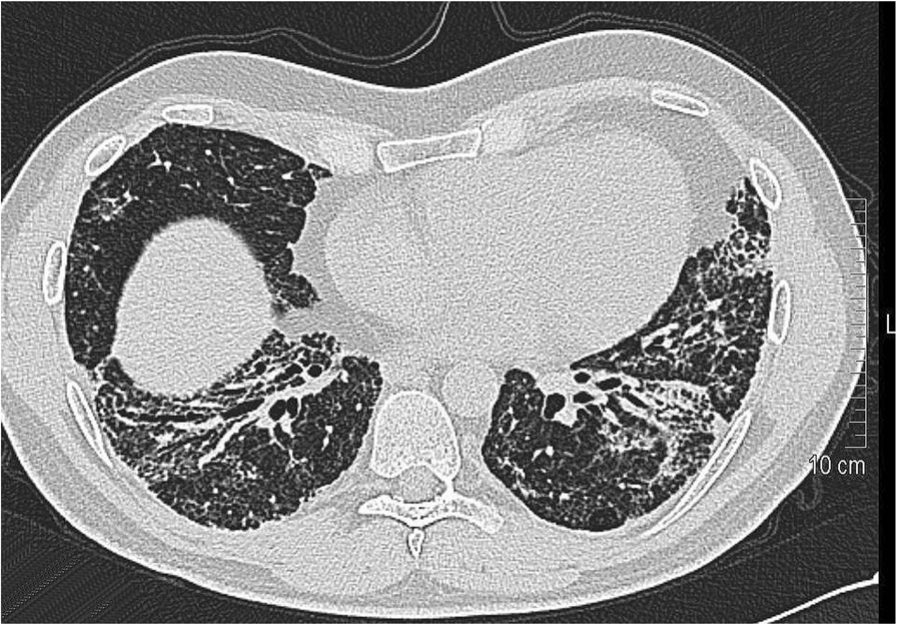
Chest Computed tomography performed after third cycle of chemotherapy showing diffuse interstitial infiltrates, bilaterally, consistent with Bleomycin induced pneumonitis.
